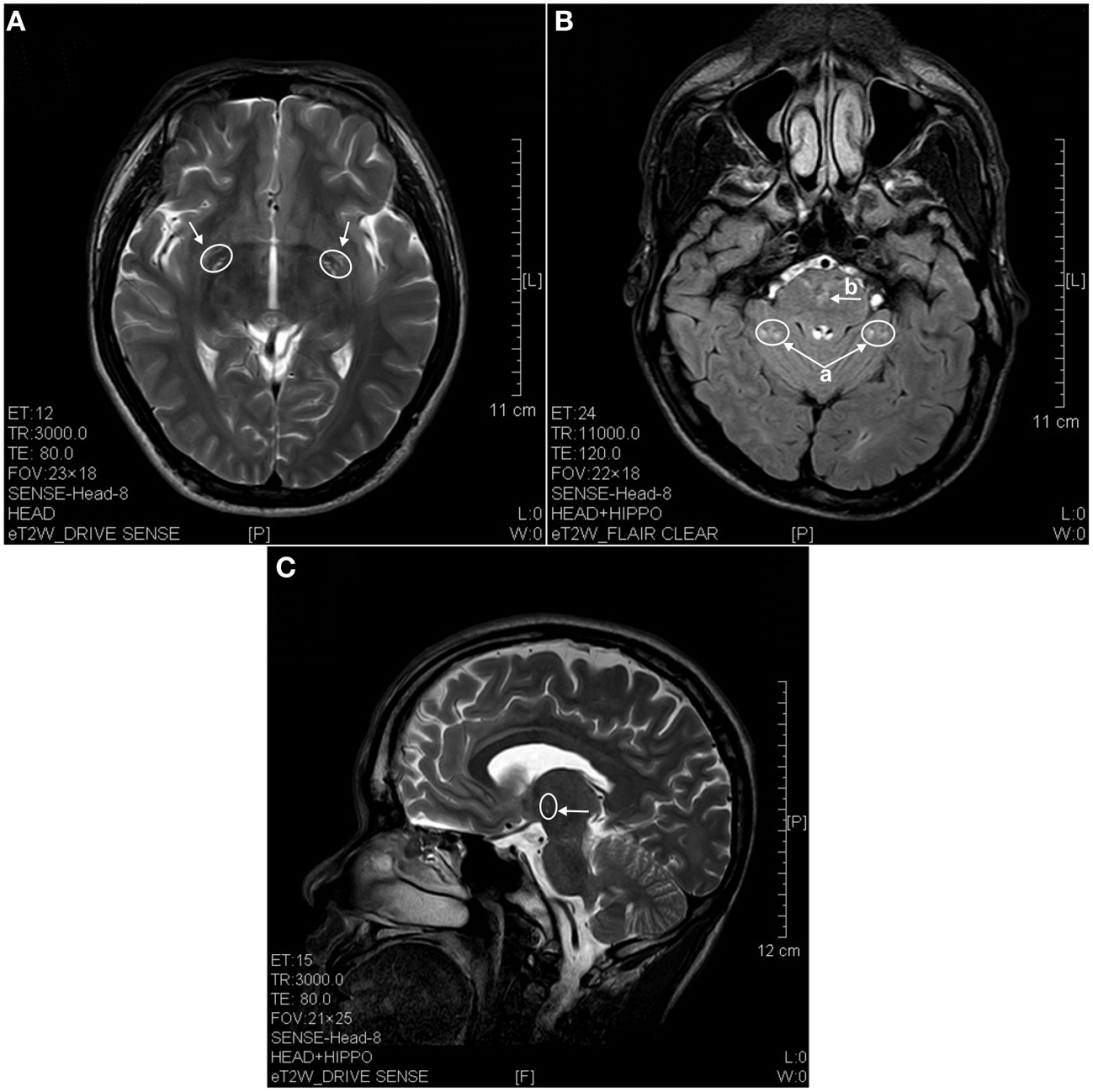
MRI images of the brains of a 4 years ketamine addicts. (A) T2 image of a horizontal brain section showing degenerative hyperintense spots in basal forebrain (arrow). (B) T2 image of a horizontal section showing hyperintense degeneration in cerebellum (a) and in pons (b). (C) T2 image of a sagittal section showing degeneration spots in diencephalon (thalamus).
